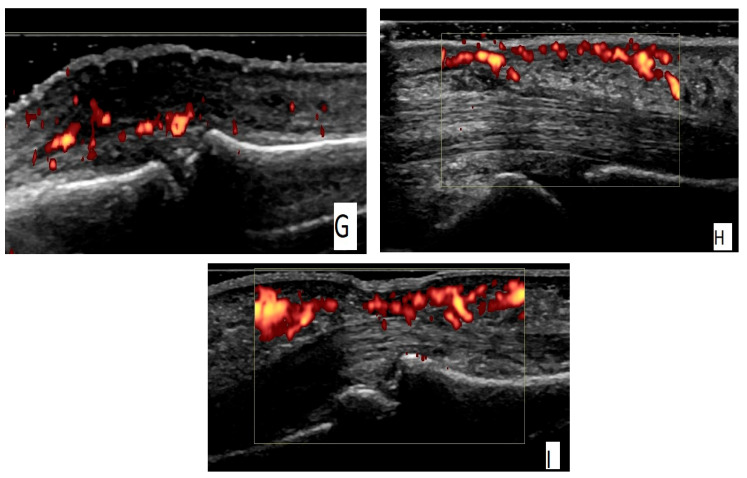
(A) An ulnar longitudinal scan of the 6th extensor compartment of the wrist (the tendon of extensor carpi ulnaris—ECU) in an RA patient. Power Doppler positive (PD) tenosynovitis of ECU tendon. (B) A transverse scan of the 6th extensor compartment of the wrist (the tendon of extensor carpi ulnaris—ECU) in an RA patient. PD-positive tenosynovitis of ECU tendon. (C) A palmar longitudinal scan of an MCP joint in an RA patient. Tenosynovitis of the flexor tendon of the finger with a positive PD signal. (D) A palmar transverse scan of an MCP joint in an RA patient. Tenosynovitis of the flexor tendon of the finger with a positive PD signal. (E) A dorsal longitudinal scan of an MCP joint in an RA patient. Grade 3 synovitis on a grayscale (GS). (F) A dorsal longitudinal scan of an MCP joint in an RA patient. PD synovitis grade 3. (G) A dorsal longitudinal scan of a PIP joint in a PsA patient. Paratenonitis of the extensor tendon of the finger with a positive PD signal. (H) A palmar longitudinal scan of an MCP joint in a PsA patient. Subcutaneous edema—pseudotenosynovitis of the finger flexor tendon with a positive PD signal. (I) A palmar longitudinal scan of a PIP joint in a PsA patient. Pseudotenosynovitis of the finger flexor tendon with a positive PD signal.
